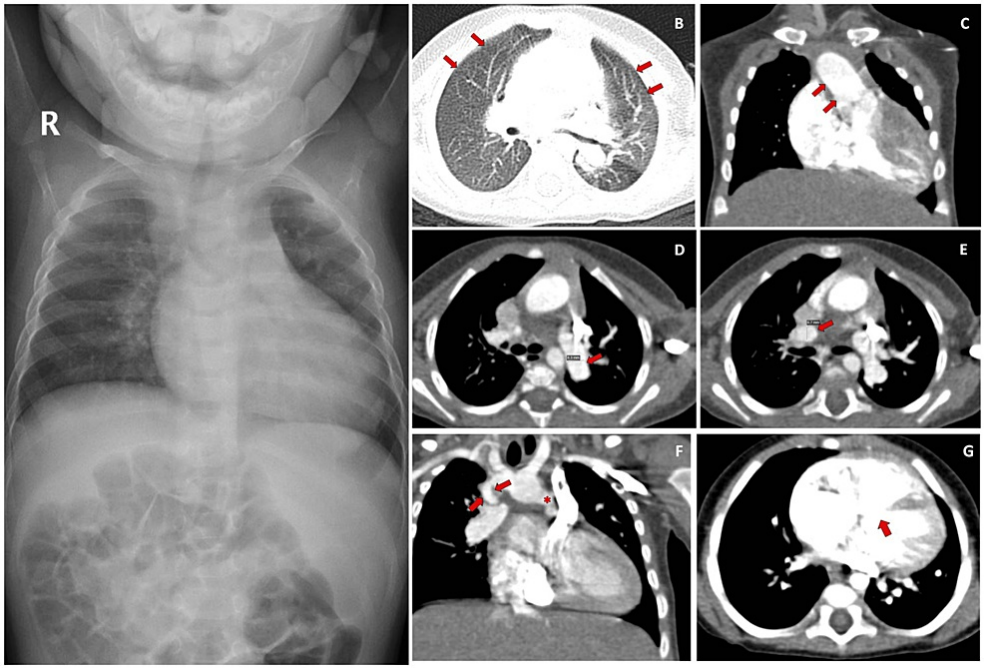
Proximal arrest of bilateral pulmonary arteries having truncus arteriosus anomaly with anomalous arterial channels from pulmonary artery and aorta supplying bilateral lungs. An 8-month-old male child was being evaluated for difficulty of breathing. As a routine part of protocol, Chest X-Ray was ordered which showed a widened mediastinum. A Chest CECT was ordered for further evaluation. The findings of Chest CECT as described below. 3A: Chest X-Ray showing widened mediastinum. 3B: Chest CT scan showing bilateral pulmonary plethora (red arrows). 3C: Chest CECT showing a common trunk (red arrows) connected with the aorta and pulmonary vasculature. 3D: Chest CECT demonstrating a hypoplastic left pulmonary artery (red arrow), 3E: Chest CECT demonstrating a hypoplastic right pulmonary artery (red arrow), 3F: Chest CECT demonstrating a right pulmonary artery arising from the brachiocephalic trunk (arising inferiorly) (red arrows) and small arterial channel (forming the left PA) seen arising from the aorta and supplying the left lung (asterisk). 3G: Chest CECT demonstrating a sub-aortic ventricular septal defect. PA: Pulmonary Artery, CECT: Contrast Enhanced Computed Tomography
